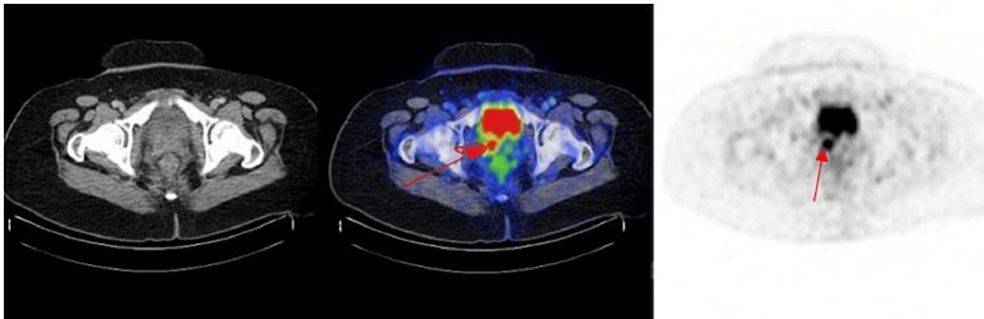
PET-CT shows an FDG-avid nodular lesion along the right anterolateral aspect of the upper third of the vagina concerning for disease recurrence; no FDG-avid disease was seen elsewhere; however, a biopsy of this nodular area showed vaginal tissue with mild chronic inflammation and fibrosis; no in situ or invasive carcinoma was seen PET: positron emission tomography; FDG: fluorodeoxyglucose
